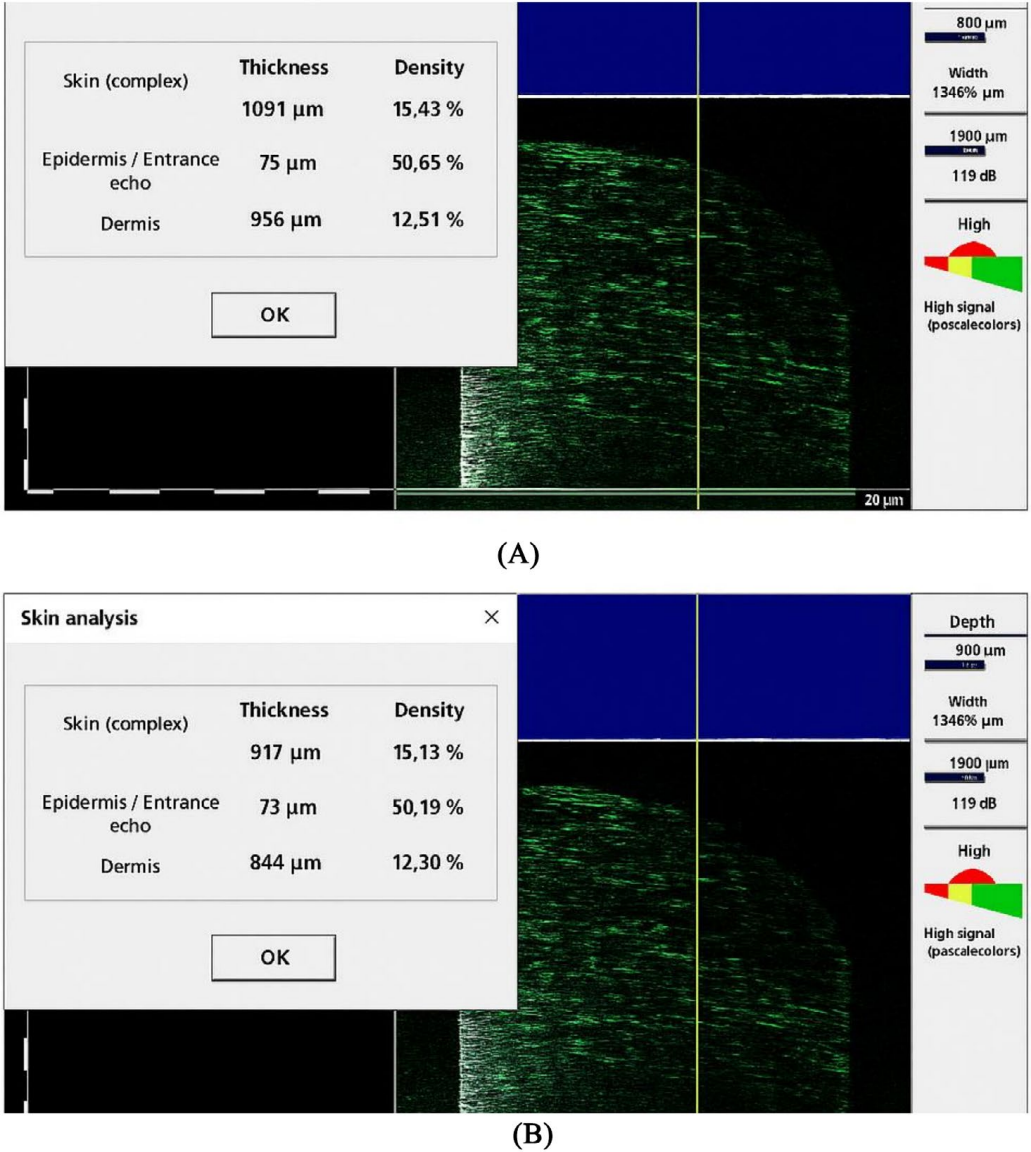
Sonographic evaluation of skin thickness and density. (A) Baseline ultrasound showing reduced dermal and epidermal thickness and lower density. (B) Post‐treatment ultrasound at 3 months demonstrating increased thickness and echogenicity, indicating tissue remodeling and regeneration.
